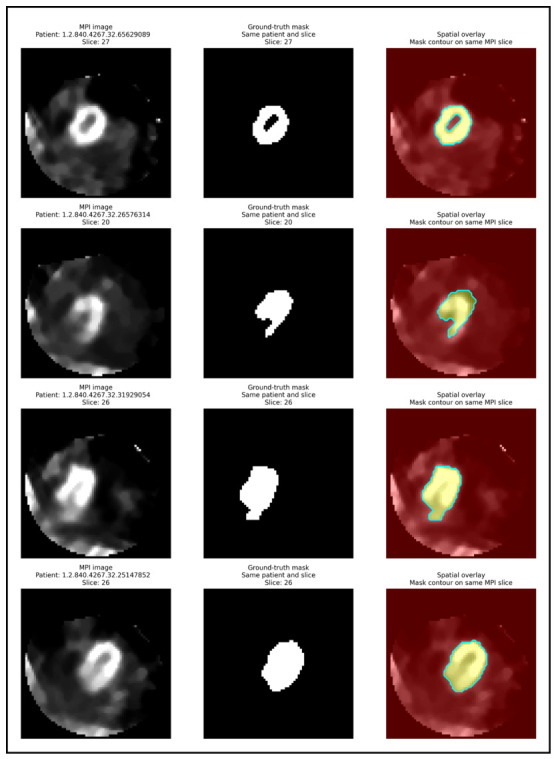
Representative myocardial perfusion scintigraphy images, ground-truth myocardium masks, and spatial overlays. Each row shows one representative patient slice from the public MPS dataset. The first column presents the grayscale MPI image, the second column shows the corresponding binary ground-truth myocardium mask, and the third column presents the spatial overlay of the mask on the same MPI slice. In the overlay panels, the red background represents the MPI slice intensity map, the yellow region indicates the myocardium mask area, and the cyan contour shows the boundary of the ground-truth myocardium mask.
